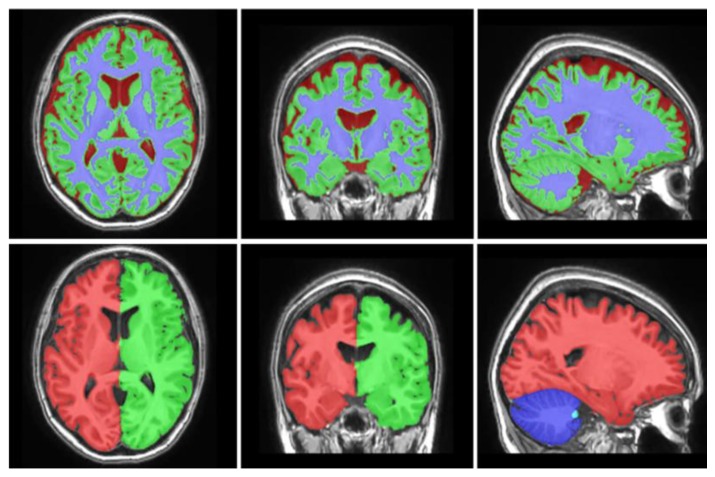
Brain gray and white matter discrimination and macrostructures with automated magnetic resonance imaging (MRI) brain morphometry.
